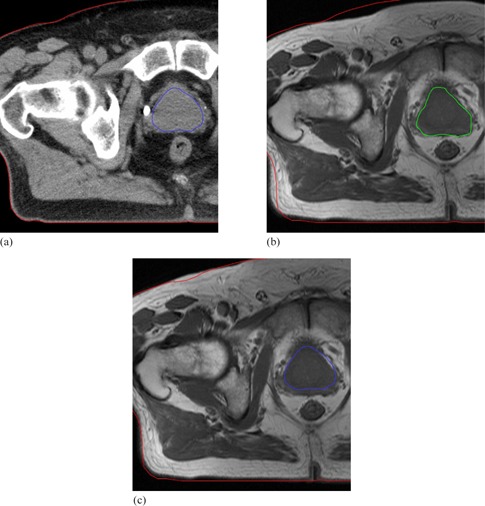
Axial slices of prostate patient whose MRI and CT images were acquired on different shaped couches: (a) CT with both the prostate and patient body outlined; (b) MRI after rigid alignment showing the MRI prostate and the CT patient contours (anatomical variation caused by the different couches is present in the bottom left hand corner); (c) CT prostate and patient contours overlaid on the MRI after deformable registration. Warping on Reveal‐MVS accounted for couch based anatomical variations but completely ignored the prostate motion.
